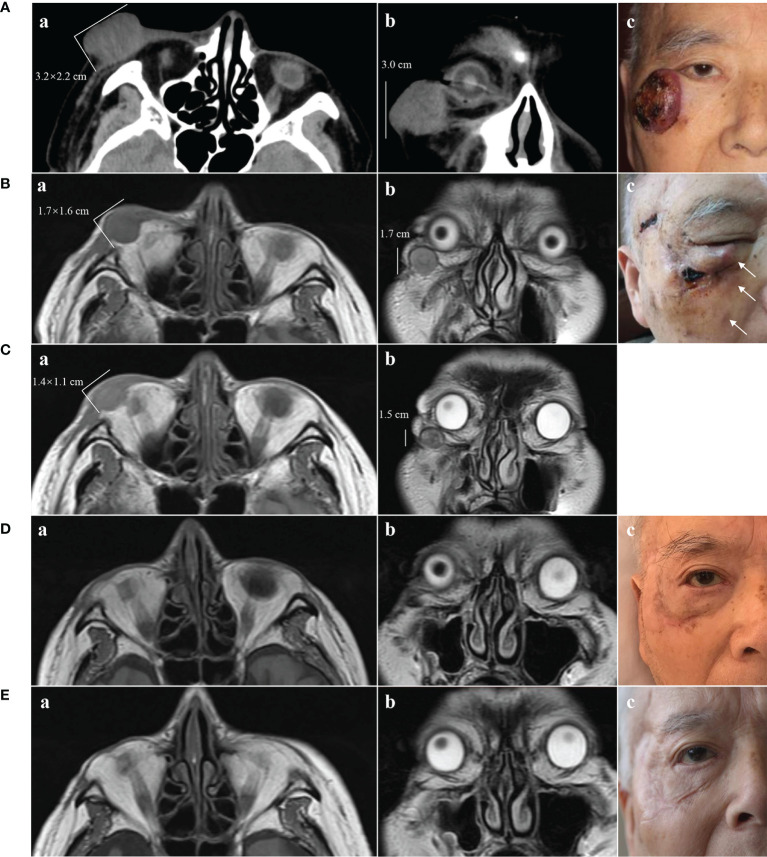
Clinical presentation of MCC and changes on imaging. (A) May 2018. A(a) and A(b) CT scans showing a tumor of 3.2 × 2.2 × 3.0 cm without destruction of bone. A(c) Solitary violet-colored nodule of the right lower eyelid with pigmentation and an irregular ulcer in the center. (B) June 2018. B(a) and B(b) MRI showing a tumor of 1.7 × 1.6 × 1.7 cm. B(c) Recurrence of the MCC. The white arrows show three hard, subcutaneous nodules. (C) July 2018, 2 weeks after treatment with apatinib. C(a) and C(b) MRI showing regression of the MCC (1.4 × 1.1 × 1.5 cm). (D) November 2018, 4 months after treatment with apatinib. D(a) and D(b) MRI showing MCC disappearance with a favorable response to apatinib. D(c) Only a scar with pigmentation was observed. (E) November 2019, 17 months after treatment with apatinib. E(a) and E(b) MRI showing no sign of recurrence. E(c) The pigmentation gradually subsided, leaving a pink scar.
